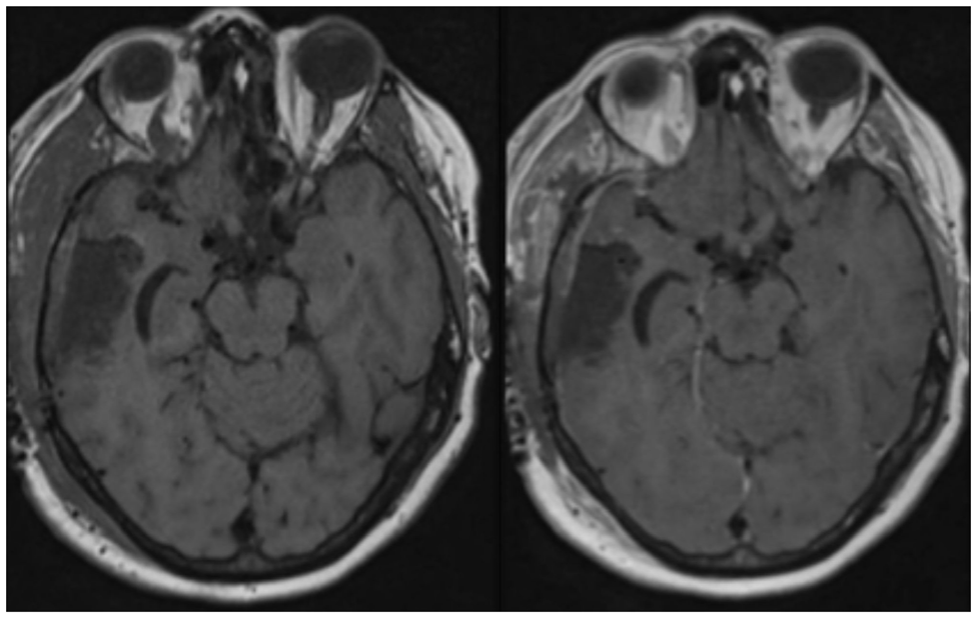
Day 1 post-operative axial pre- and post-contrast T1-weighted MRI showing no significant residual tumour post-craniotomy and resection.
